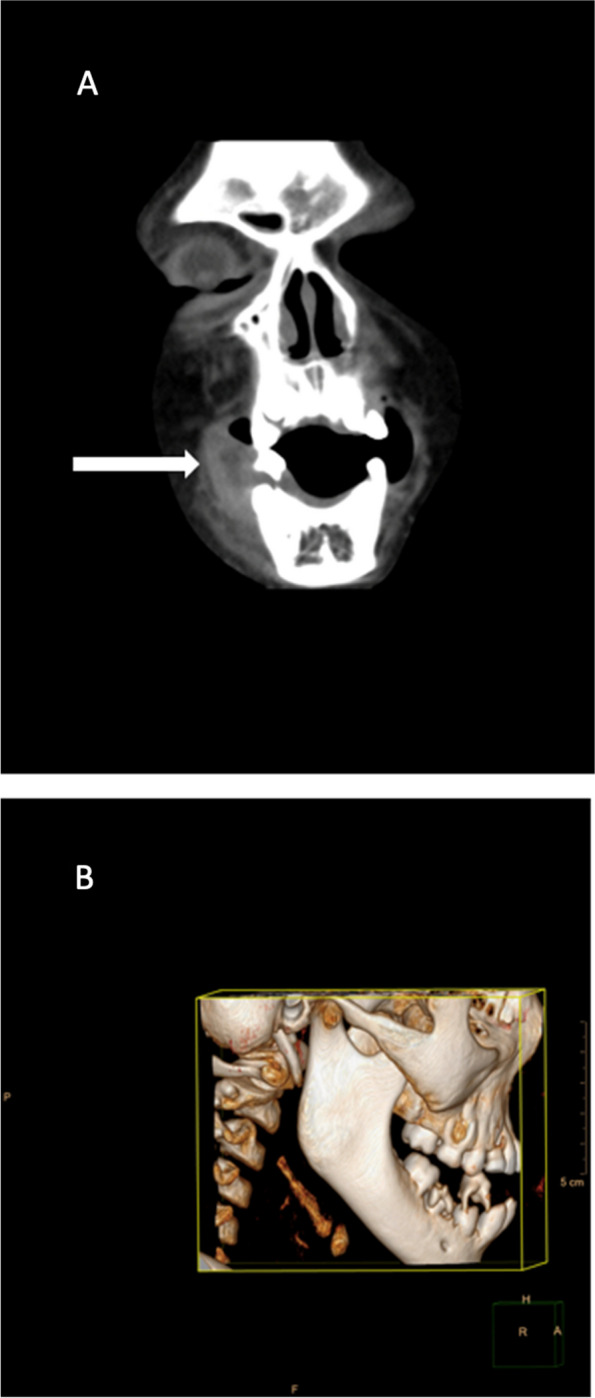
Un-contrasted CT scan of patient’s mandible: shows right peri-mandibular soft tissue swelling with a central low density suggestive of an inflammatory mass with central necrosis and no signs of destructive bone changes
